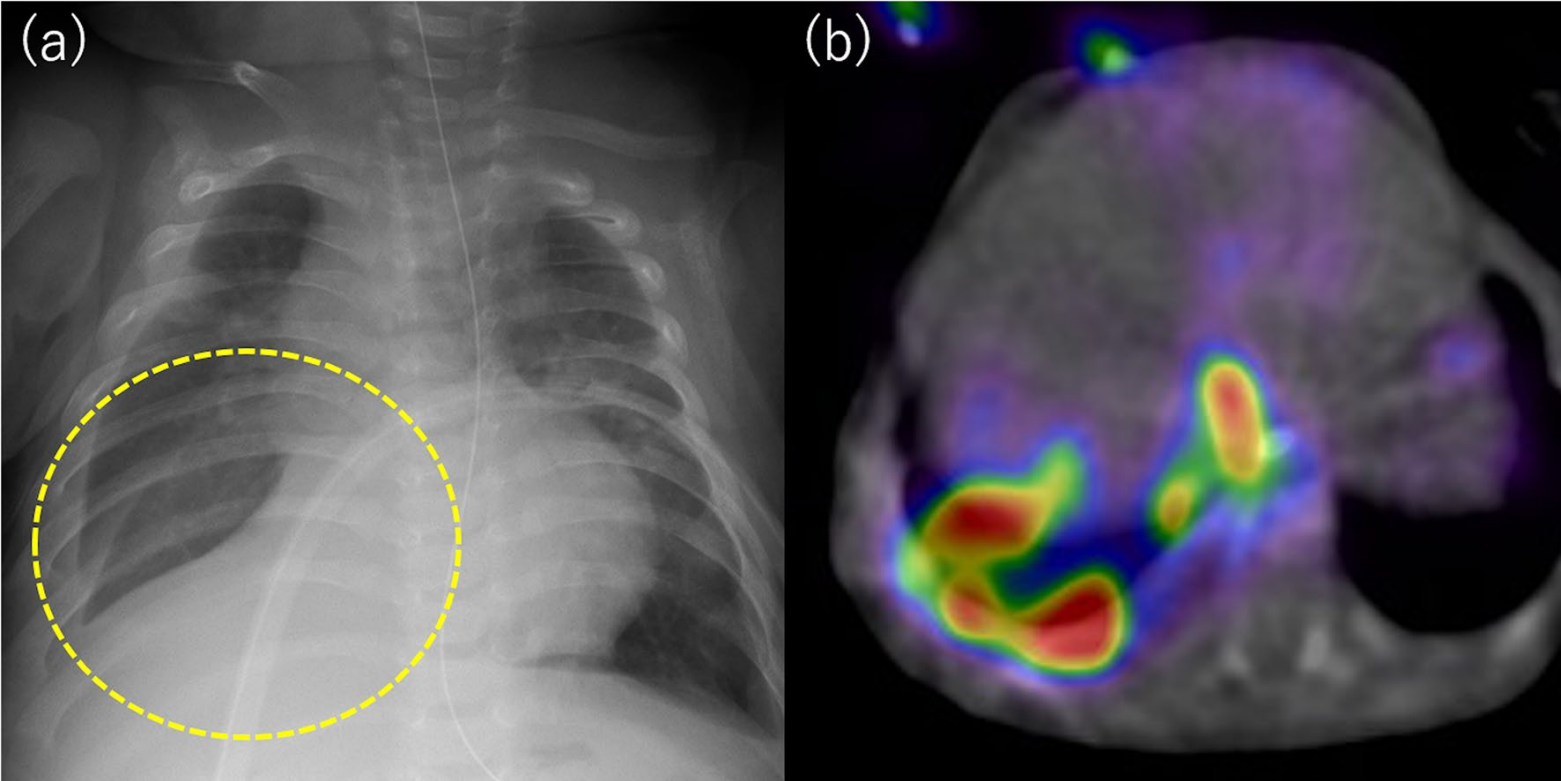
a A chest radiograph showed a right-sided hydrothorax. b Preoperative scintigraphy demonstrating the radioactive dialysate in the thoracic cavity confirming a PPC
